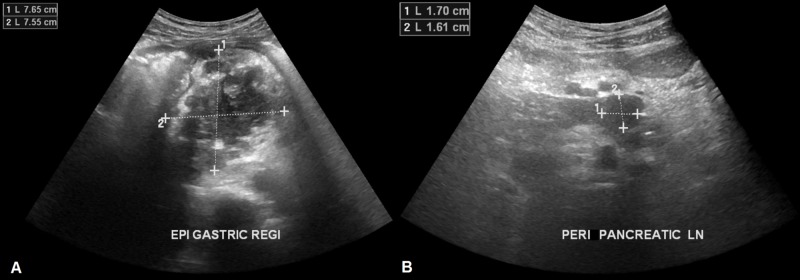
Images of abdominal ultrasonography (A) A large (7.6 cm × 7.7 cm) heterogeneous lesion is seen arising from the pancreas. (B) A peri-pancreatic lymph node is visible measuring 1.7 cm × 1.6 cm in size.
